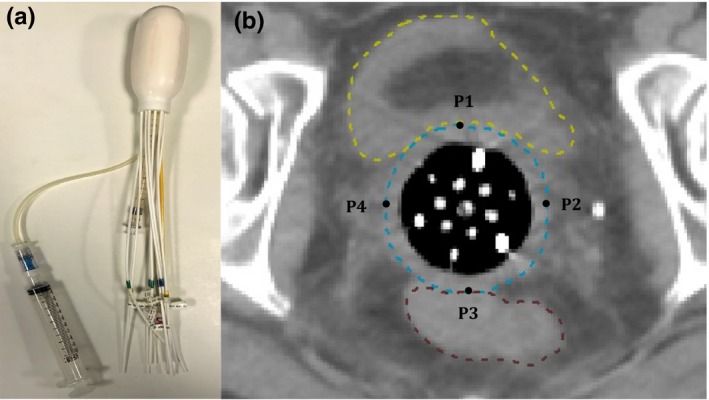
Introduction of the Capri applicator: (a) picture of a 13‐catheter Capri applicator with a syringe connected; (b) an axial CT image of a Capri applicator in patient. Contours include the bladder, the rectum, and the envelope of Capri with 5 mm expansion. Prescription points (P1–P4) placed onto the envelope are used in plan optimization. There are a total of 16 prescription points (P1–P16) for each MC re‐calculated plan.
